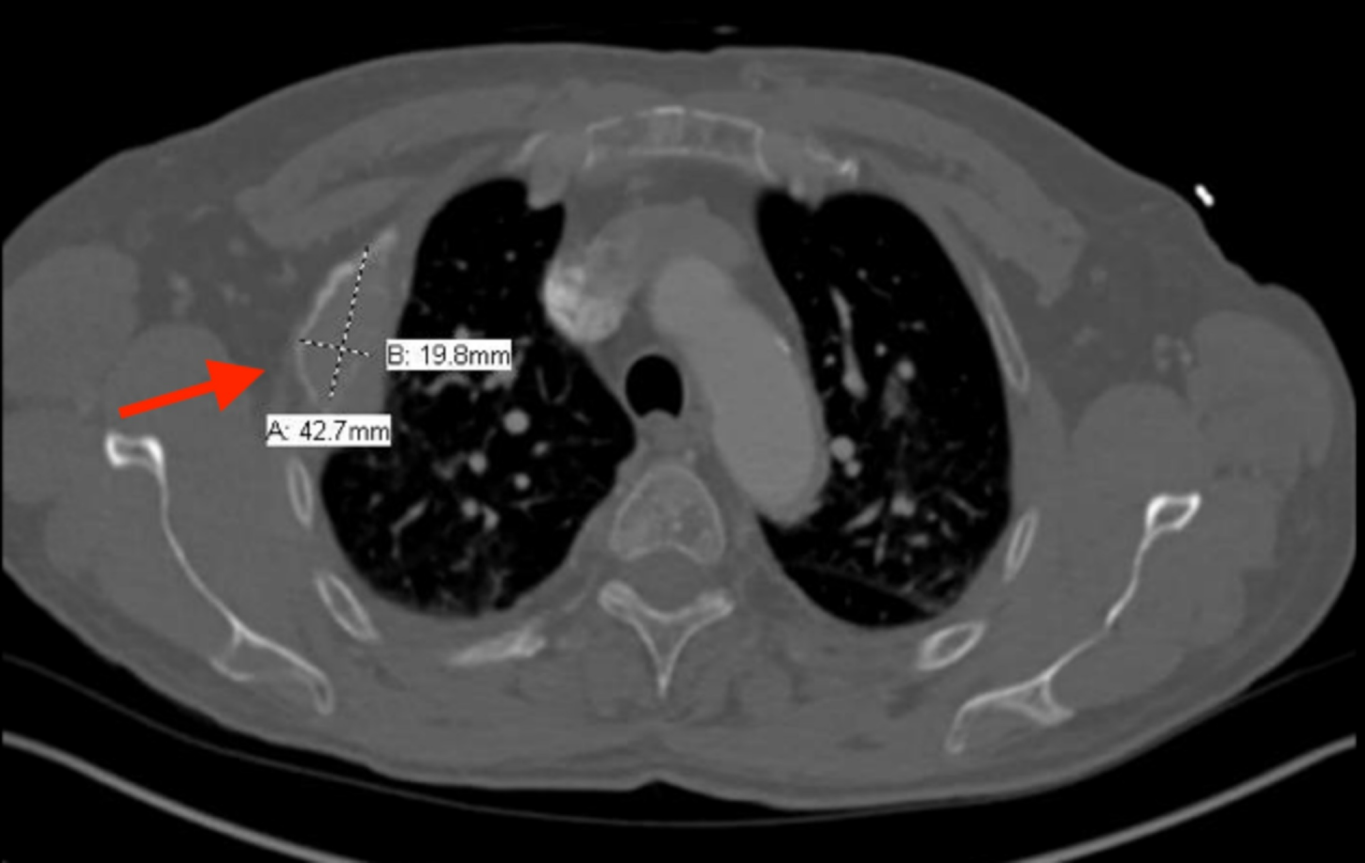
Non-contrast CT scan of the chest revealing an expansile lytic lesion involving the right rib with soft tissue involvement (red arrow) concerning for malignancy CT: Computed tomography
